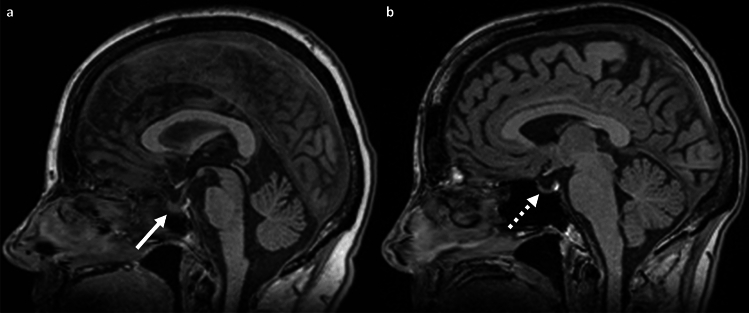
Variations of the pituitary gland on midline sagittal reconstructed T1-weighted images; (a) 29-year-old male participant (BMI 25.4 kg/m^2^) with normal height of the pituitary gland (white arrow). (b) 32-year-old male participant with empty sella phenomenon (dotted white arrow).
